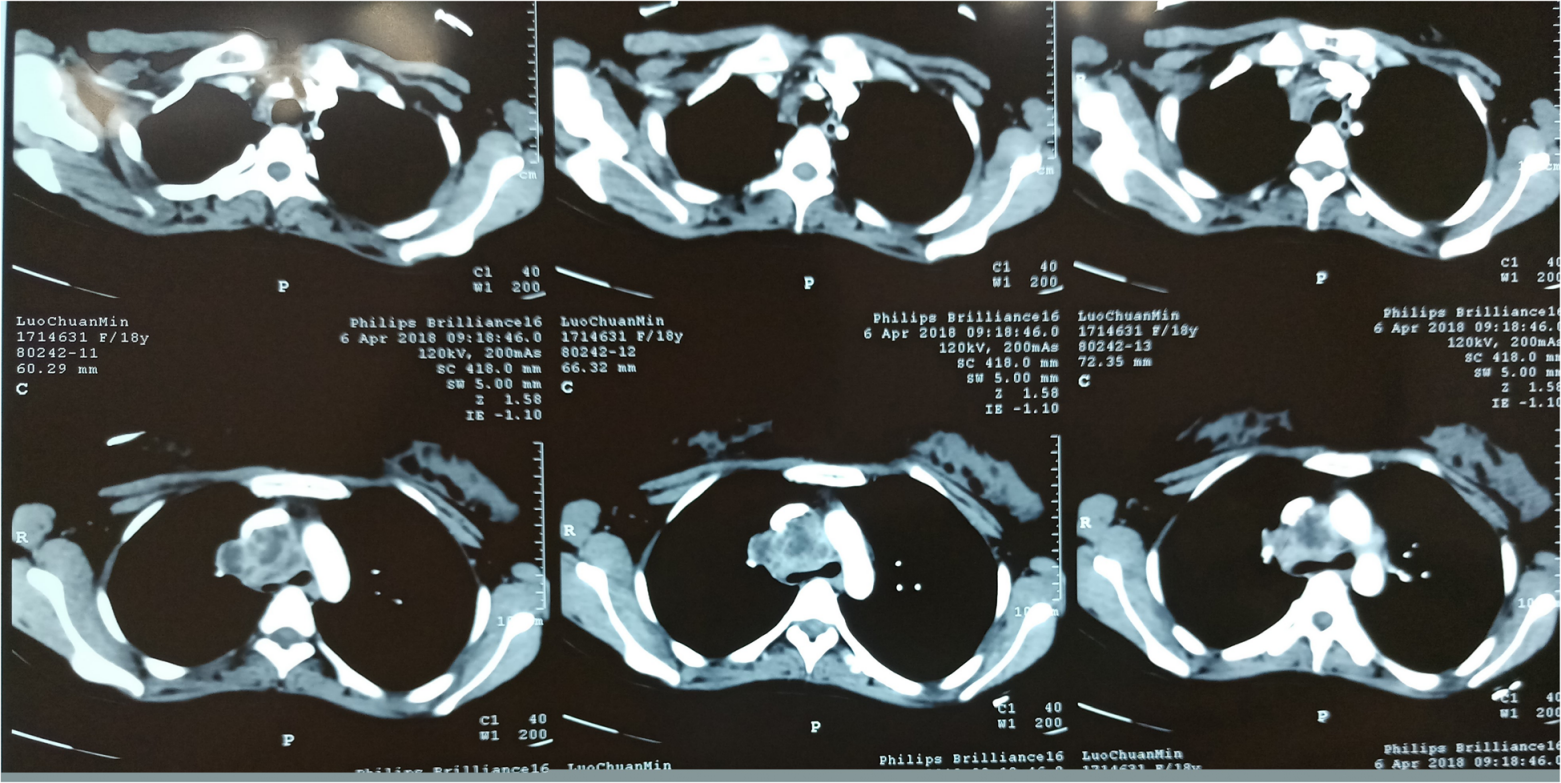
Chest CT reexamination on April 6, 2018
